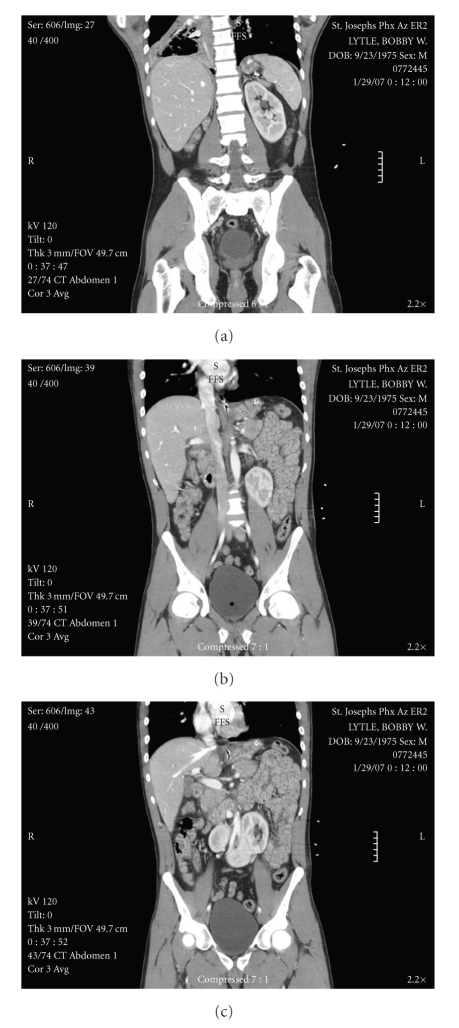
This is a series of coronal sections from a computed tomography (CT) scan of the abdomen and pelvis, done as a screening scan for abdominal trauma when the donor was admitted to the hospital. Image (a) shows the left kidney in a normal anatomic position. Image (b) shows the inferior fusion plane. Image (c) shows the right ectopic kidney, crossing the midline across the iliac vessels.
